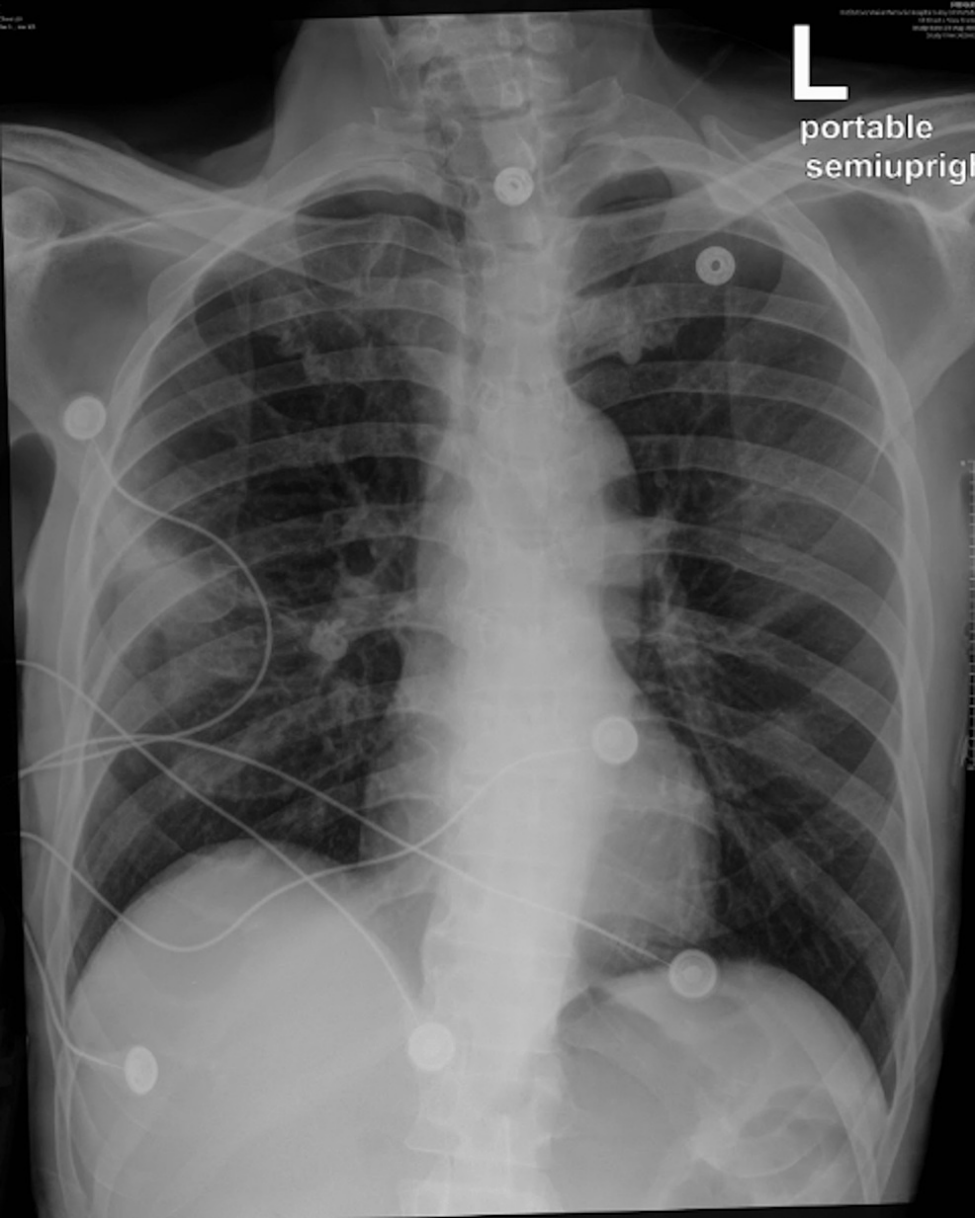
Chest X-ray post removal of the draining catheter
